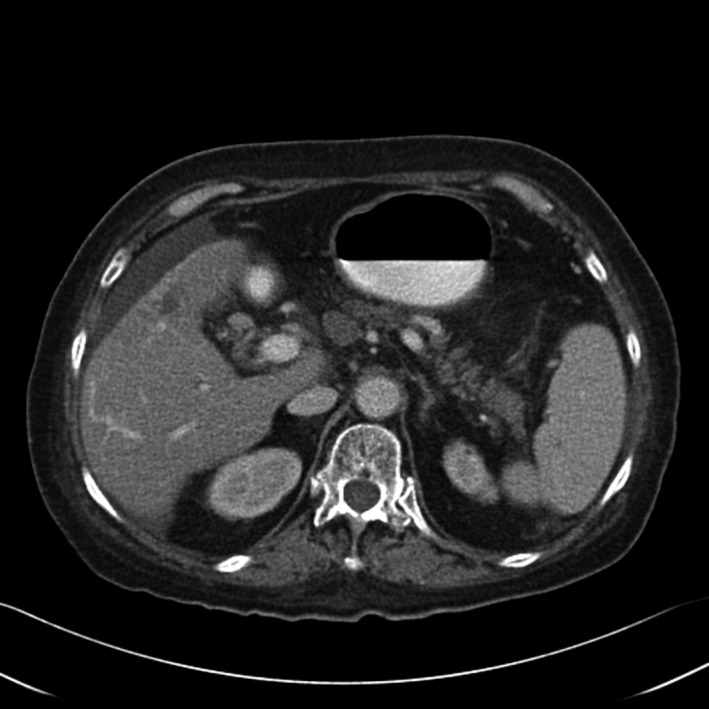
Patient 2 follow‐up CT image venous phase.
